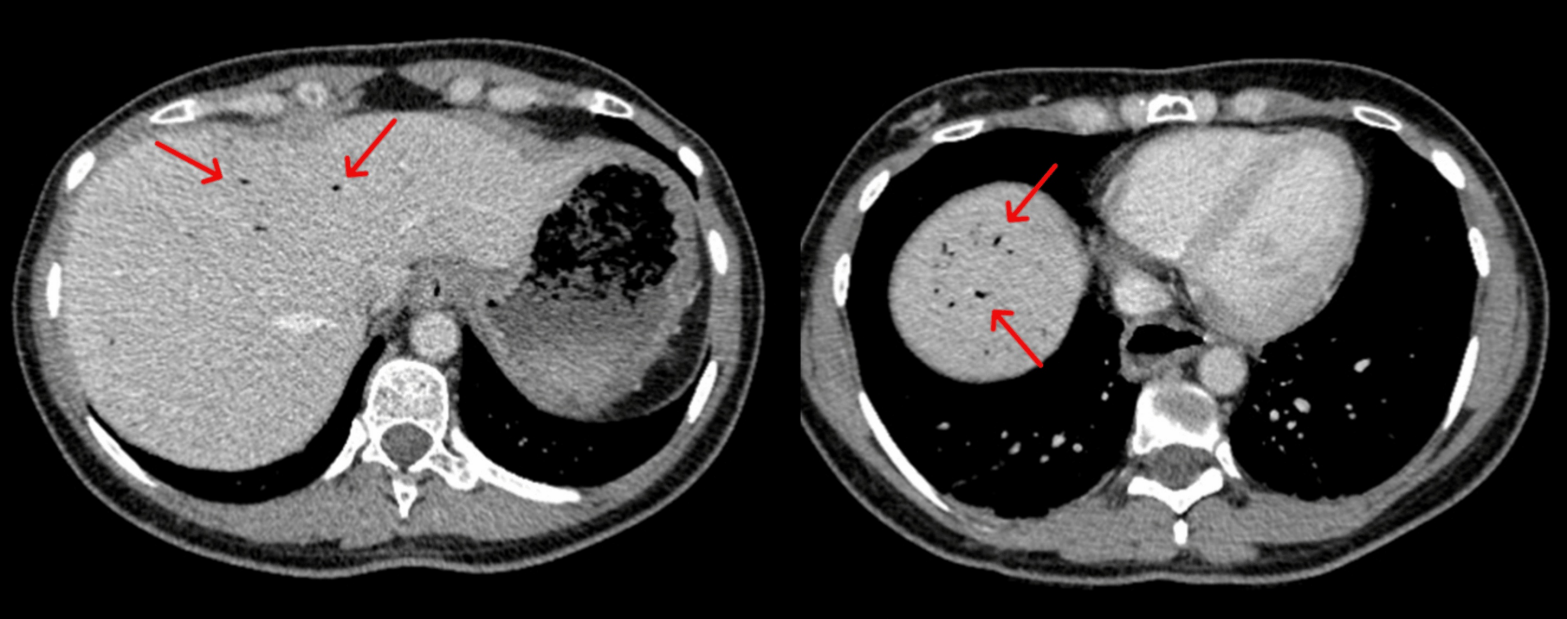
Transverse CT image showing marked locules of hepatic portal venous gas CT: computed tomography
